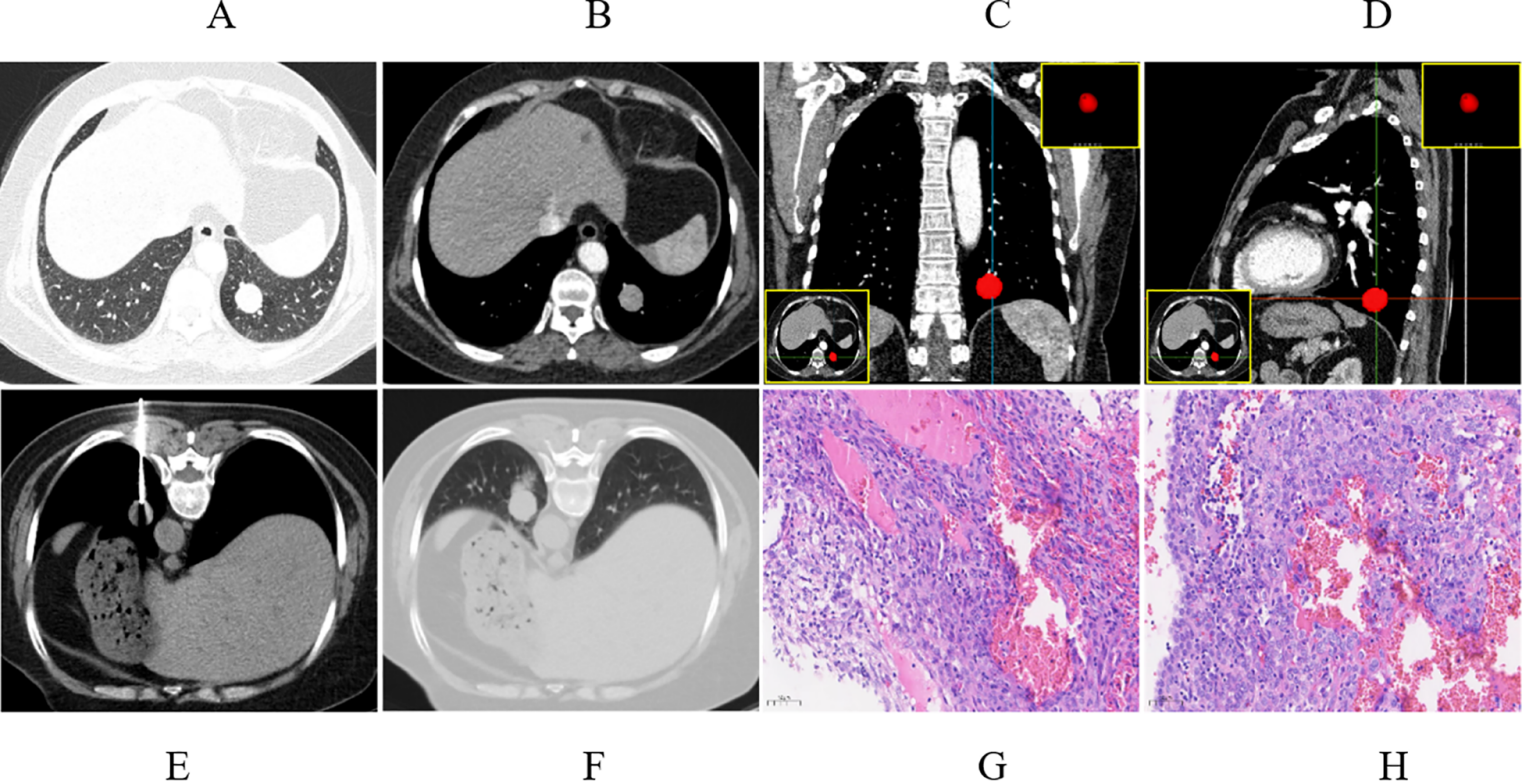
A 56-year-old female, presented with solid nodules in the left upper lobe. (A) Axial CT images of a soft tissue nodule in the posterior basal segment of the lower lobe of the left lung, measuring approximately 2.12 cm in length (lung window). (B) Significant enhancement of the lesion in the arterial phase (mediastinal window). (C, D) Coronal and sagittal CT images were utilized to precisely delineate the location of the lesions. (E) The patient underwent puncture biopsy in a prone position with dorsal entry (mediastinal window). (F) Follow-up chest CT revealed minimal bleeding in the lung tissue around the needle tract (lung window). (G) CT-guided pathological results of the puncture suggest the diagnosis of PSP (HE, 20×). (H) The pathological results post-surgical resection confirmed the diagnosis of PSP (HE, 20×).
